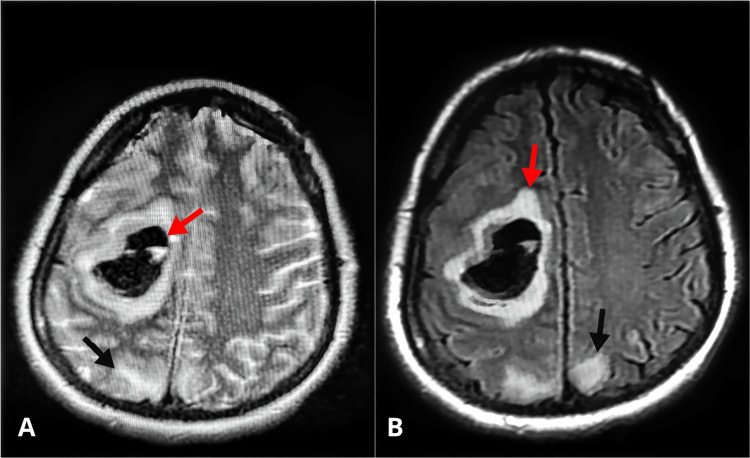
Axial T2 FSE view (A) and FLAIR view (B) Image 16/21: Red arrows indicate intracerebral hemorrhage while black arrows are suggestive of PRES FSE: Fast spin echo; FLAIR: Fluid-attenuated inversion recovery; PRES: Posterior reversible encephalopathy syndrome
